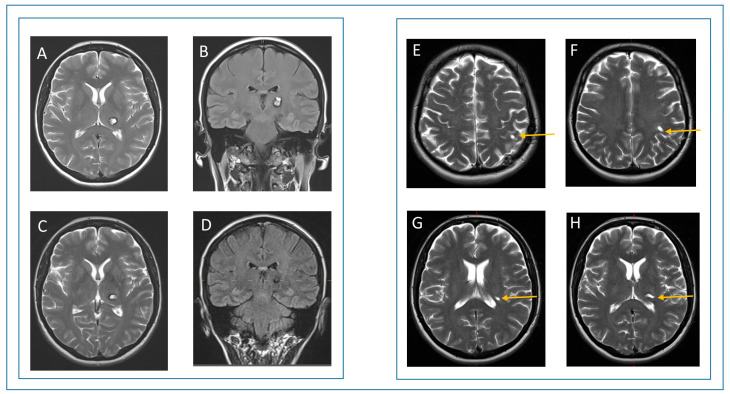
Pre- and postoperative MRI. On the left, the first row shows a cystic lesion in the left thalamus in T2 weighted sequence (A) and T2-FLAIR sequence (B). The second row shows a complete removal of the lesion. The hemosiderin ring is still slightly visible in T2-weighted sequence (C) and T2-FLAIR sequence (D). On the right, the postoperative course and size of the trajectory (yellow arrows) are shown in axial plane (E–H).
